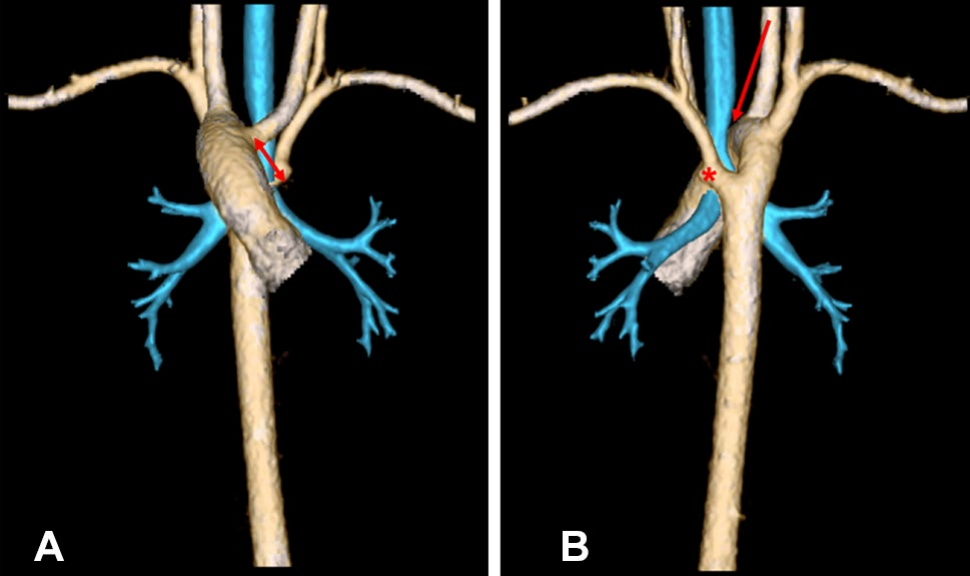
Anterior and posterior views of a right aortic arch with aberrant left subclavian (A and B). Note the significant tracheal compression (red arrow). Double red arrow: ligamentum arteriosus (not captured by CT imaging). Asterisk: Kommerell diverticulum
